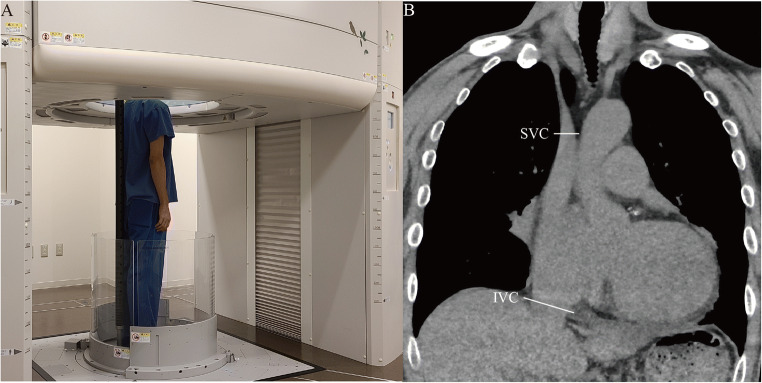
Upright computed tomography (CT) machine and measurements of the superior vena cava (SVC) and inferior vena cava (IVC) areas. A Upright CT was performed in the standing position with the subject’s arms down during deep inspiration breath-hold. The upright CT system allows up-and-down movement of a transverse 320-detector-row gantry. B Measured points on the vena cava: the SVC was measured directly below the junction of the bilateral brachiocephalic veins, and the IVC was measured at the height of the diaphragm
